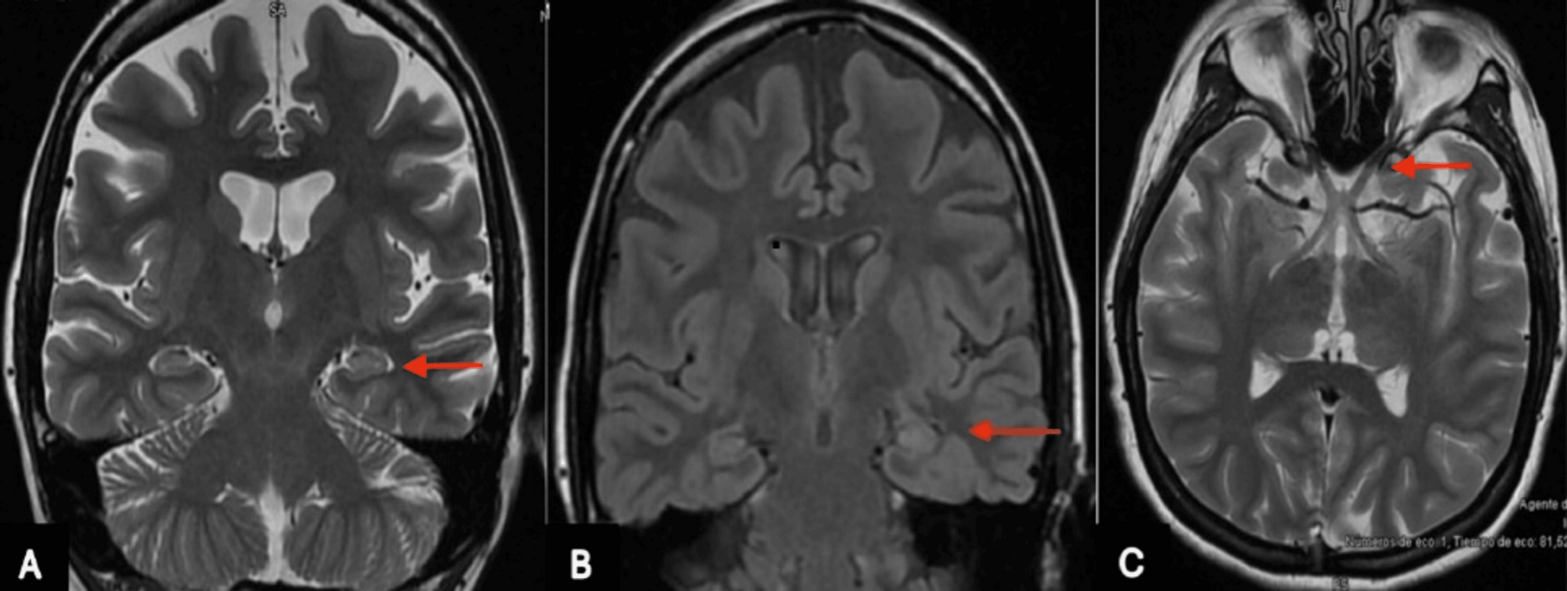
Coronal 3.0 T brain MRI (A: T2-weighted, B: FLAIR) shows a subtle increase in signal intensity in the left parahippocampal gyrus, more evident on the FLAIR sequence (red arrow), consistent with left mesial temporal sclerosis. Axial T2-weighted image (C) shows no abnormalities in the optic nerves (red arrow). FLAIR: fluid-attenuated inversion recovery
